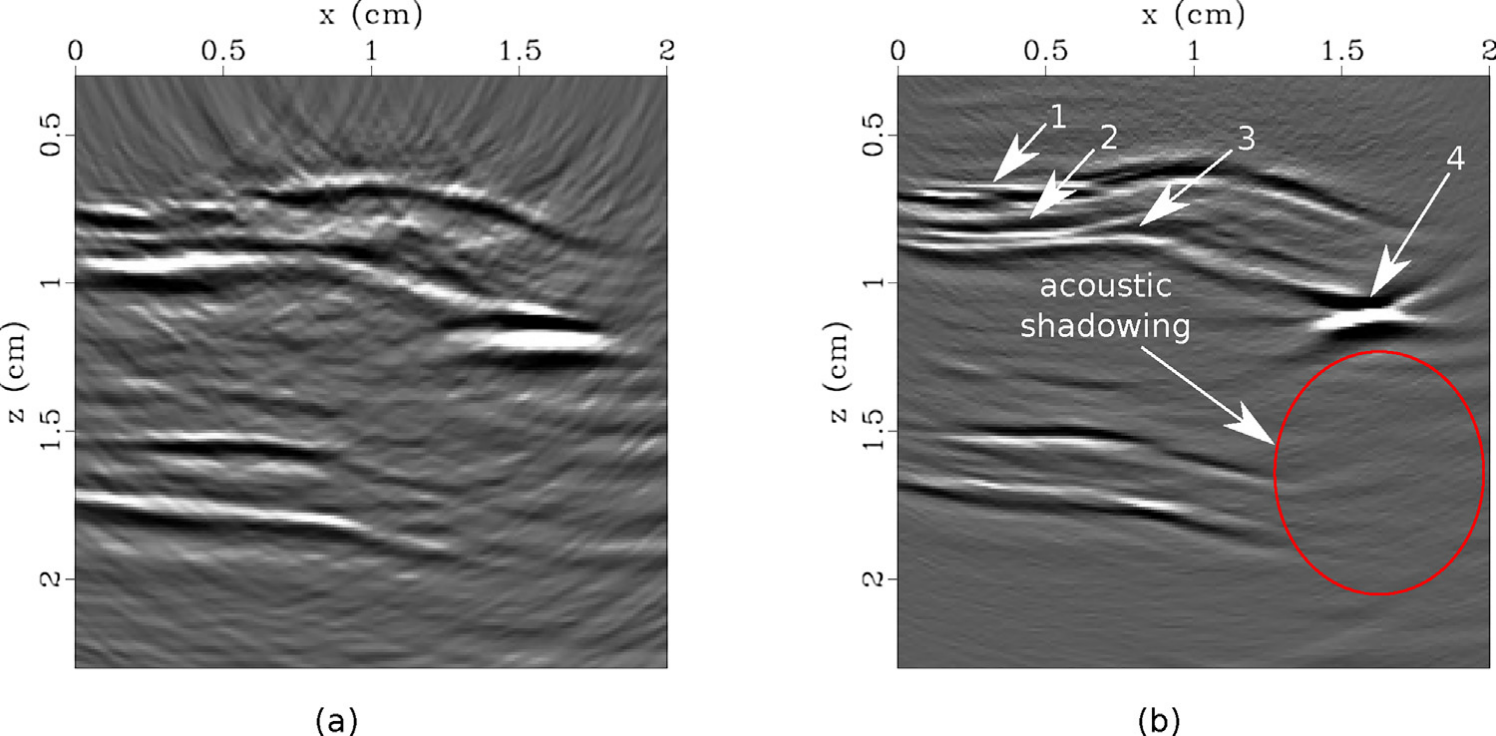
Laser-ultrasound images created with confocal (a) and nonconfocal (b) acquisition. Images are saturated to highlight the details of the artery wall. In (b), arrows 1–3 indicate the adventitia, media, and intima layers of the wall, and arrow 4 denotes calcification. Acoustic shadowing accompanies the calcification.
